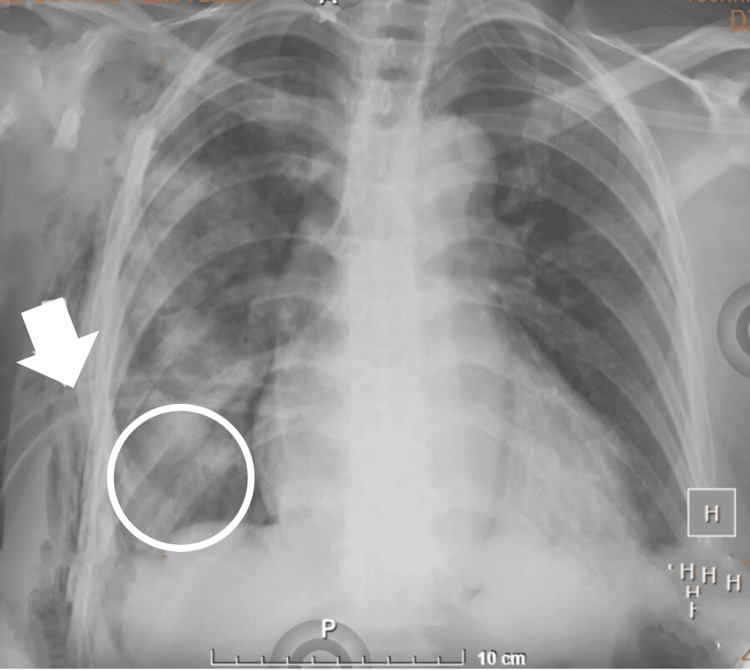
Chest X-ray after placement of an endopleural seal for drainage of massive hydrothorax Anteroposterior chest X-ray showing persistent occupation of the pleural space by fluid-dense tissue (white circle). The course of the endopleural tube is also indicated (white arrow).
